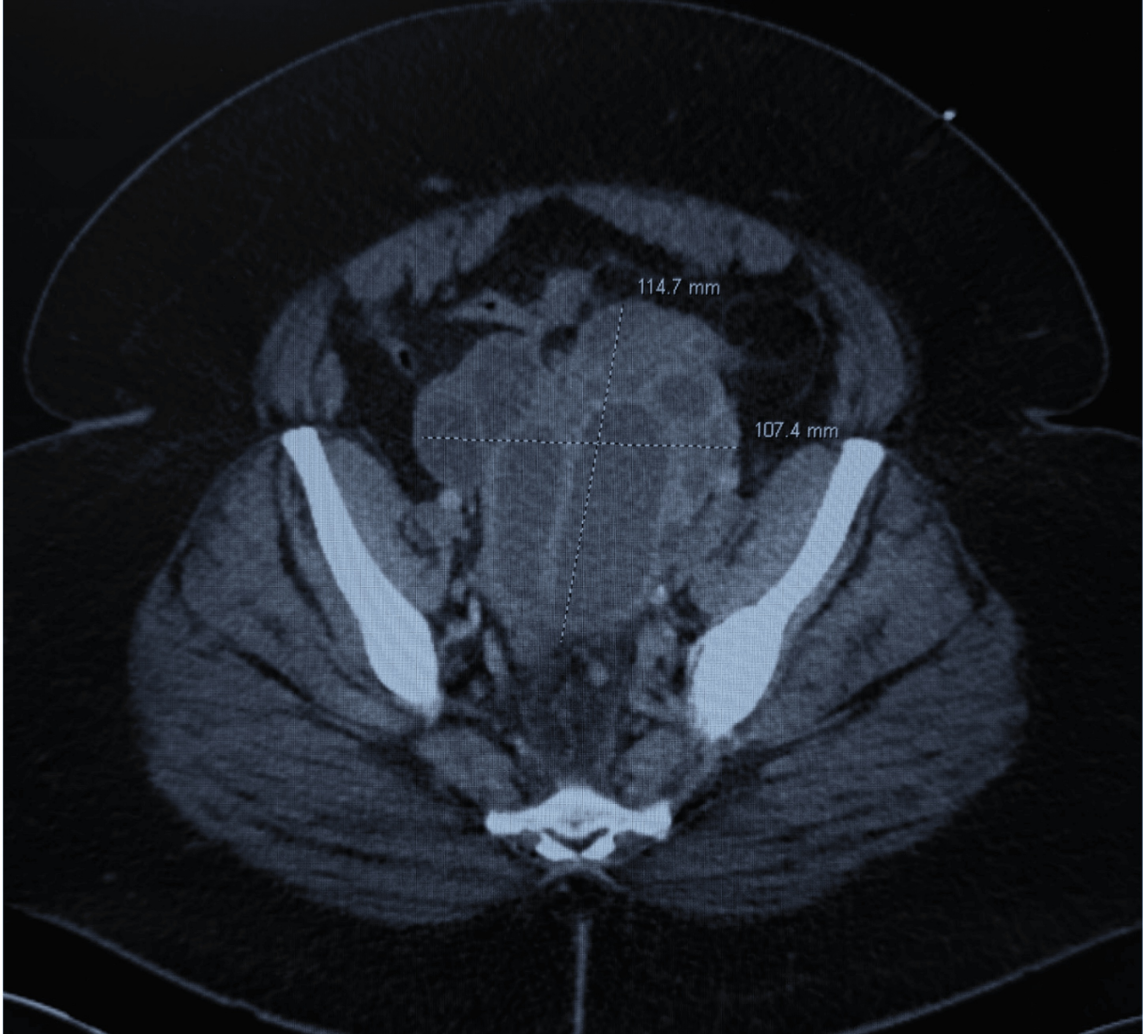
CT image of abdomen/pelvis CT abdomen/pelvis demonstrating a large complex septated mass in the pelvis containing multiple tubular structures consistent with adnexal origin.
